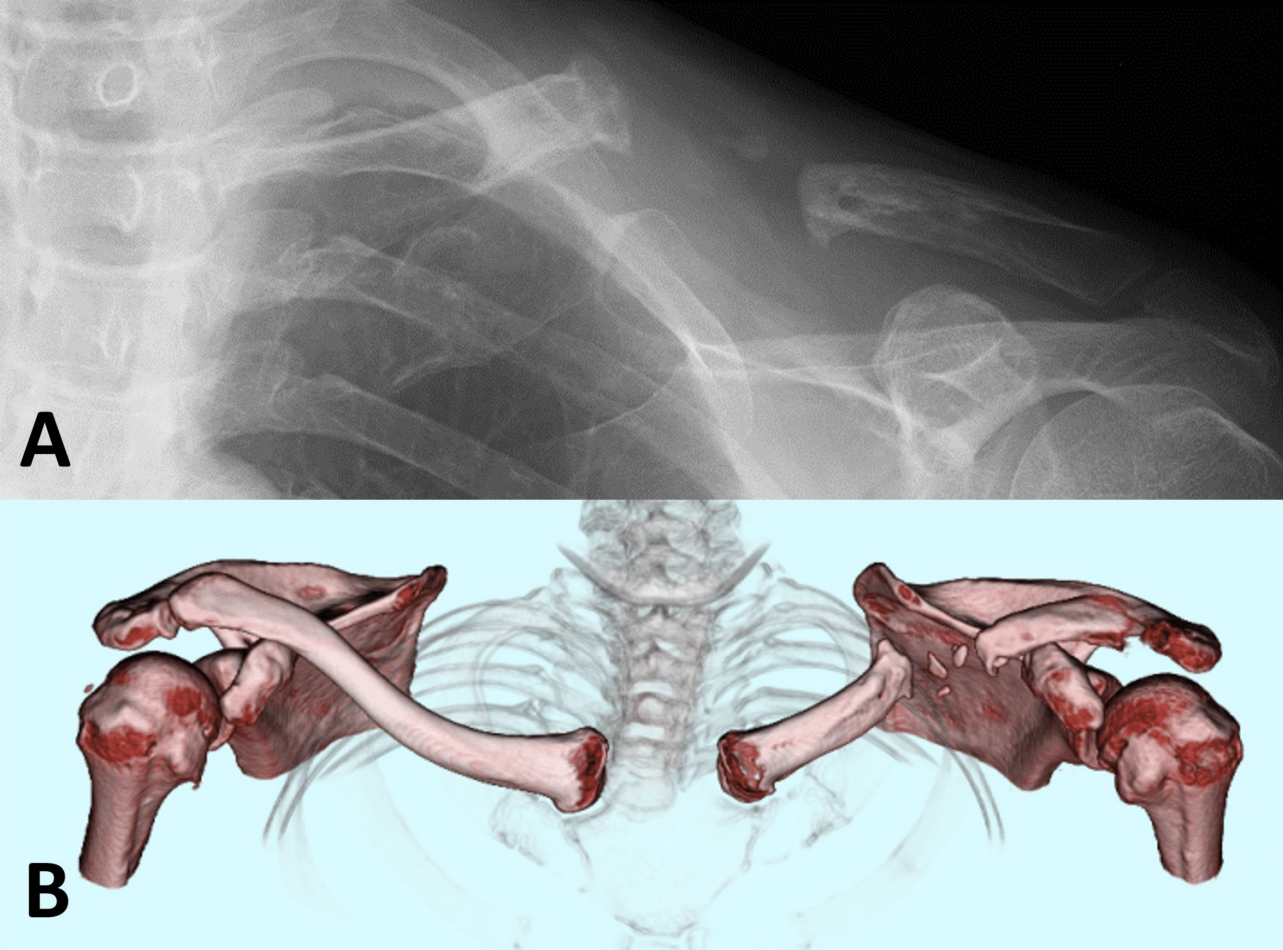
Recalcitrant nonunion X-ray imaging and three-dimensional computed tomography showed complete resorption of the bone graft and increased dissociation of the fracture fragments at three years and six months post-injury (A, B).
